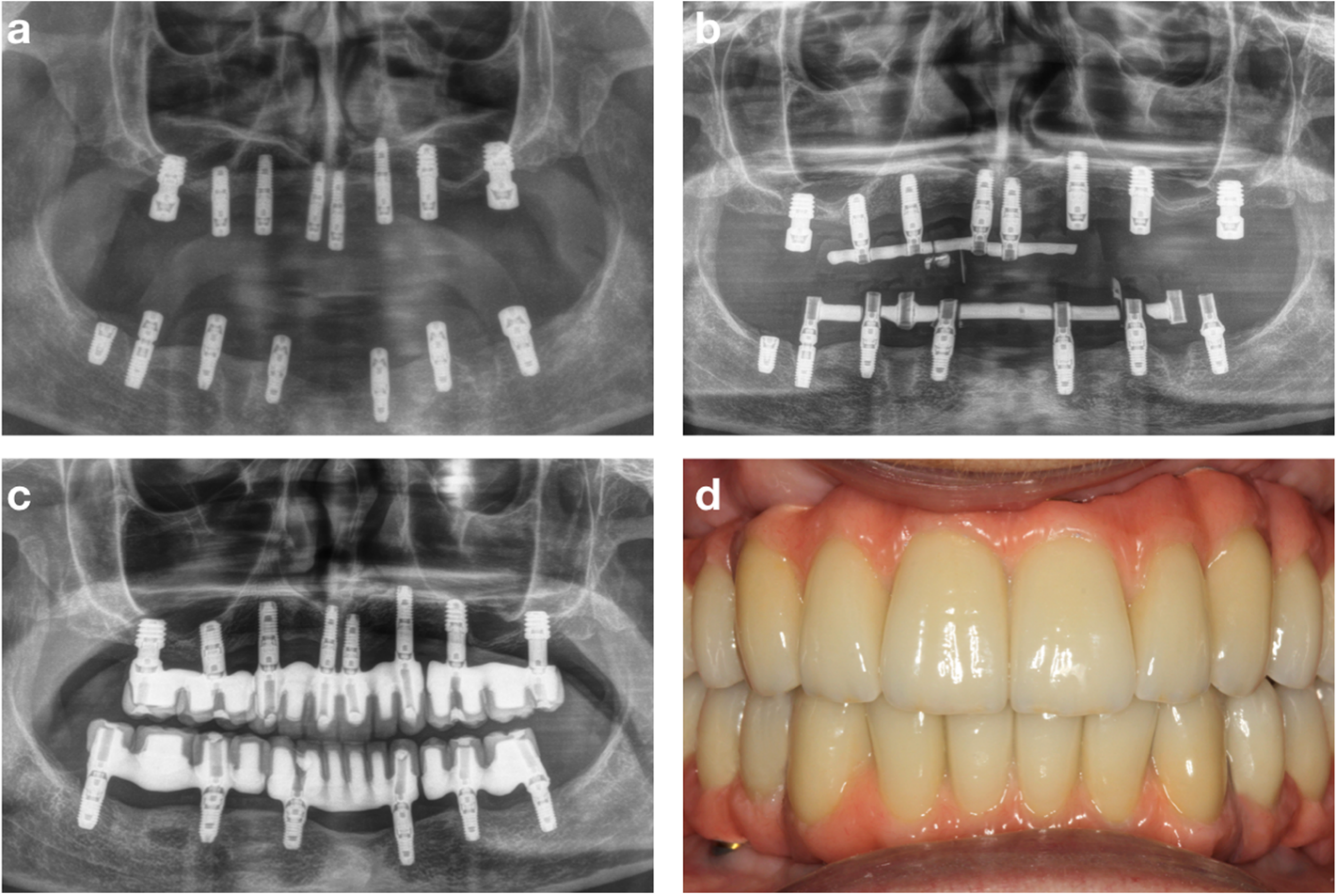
Implant placement surgery after 4 months of healing (a). Immediate loading of the new implants and the explanation of the implant at the left first molar (b). Panoramic radiograph showing the case finished with 12 months of follow-up (c). Clinical image showing the definitive screw-retained prostheses (d)
